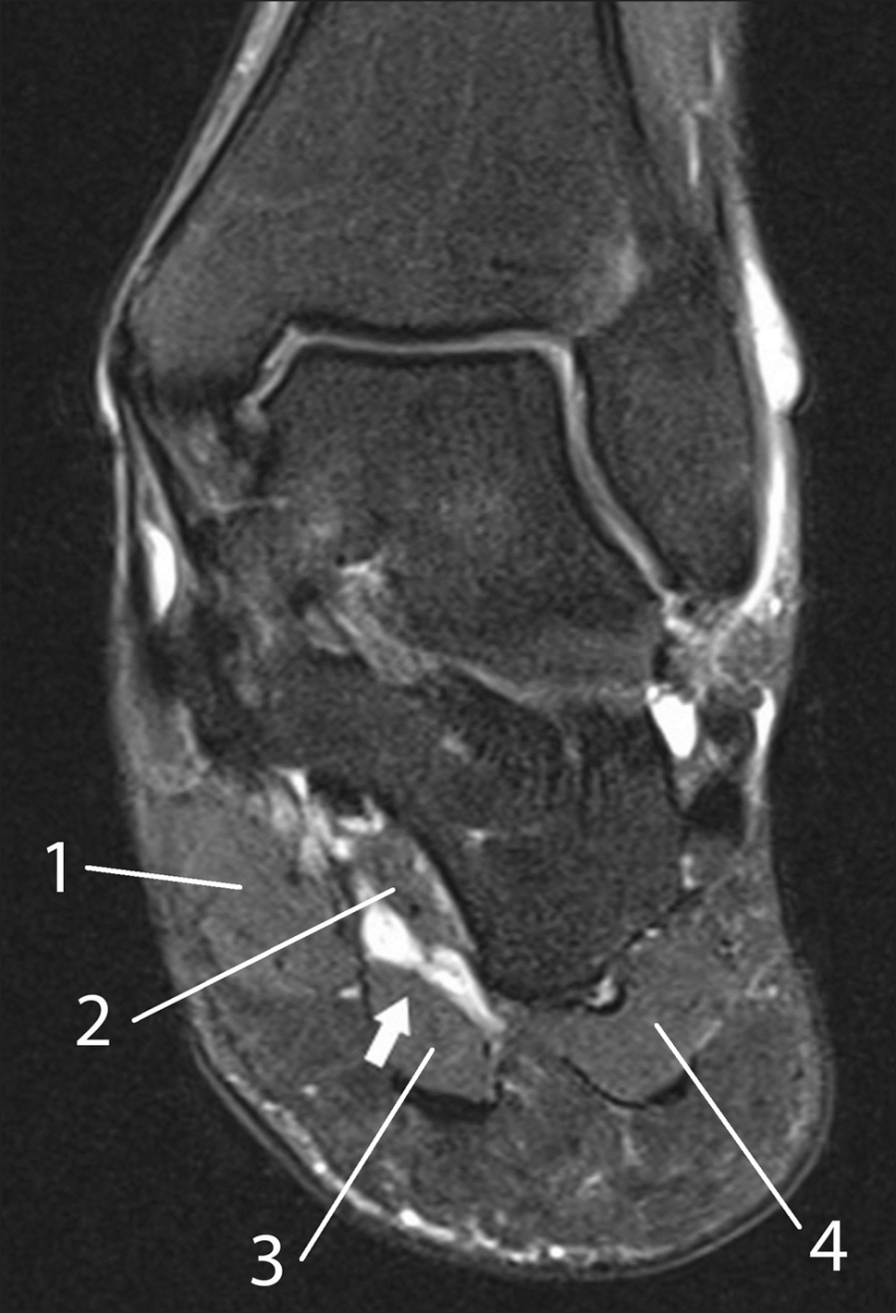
MRI of the left foot in a normal patient for comparison. Coronal reformatted T2-WI showing clearly the hyperintense medial plantar veins (arrow) in normal conditions. The veins are surrounded by the m. abductor hallucis (1) medially; the m. quadratus plantae (2) deeply from the veins; and the m. flexor digitorum brevis (3) laterally. The muscle belly of the m. abductor digiti minimi (4) is located at the lateral plantar aspect of the foot.
